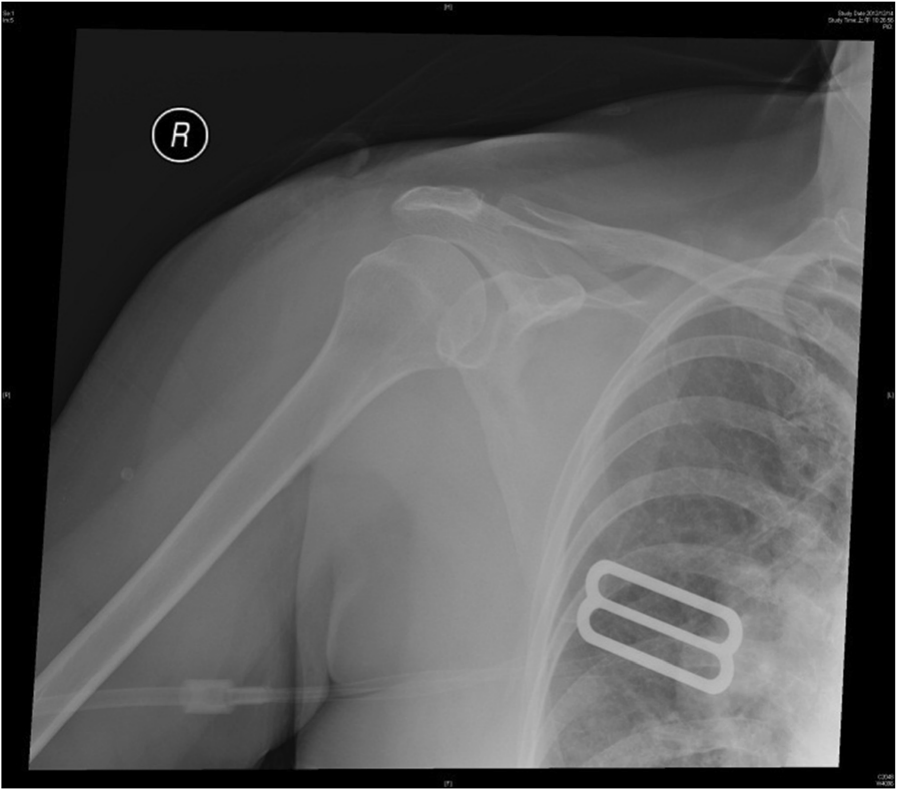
Right shoulder radiograph showing successful closed reduction.
